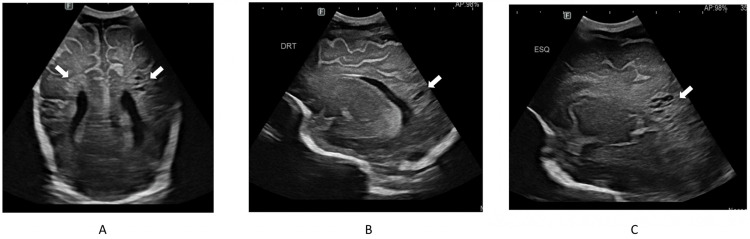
Coronal (A) and parasagittal (B,C) cranial ultrasound scans showing bilateral parieto-temporo-occipital porencephalic cysts (white arrows).
